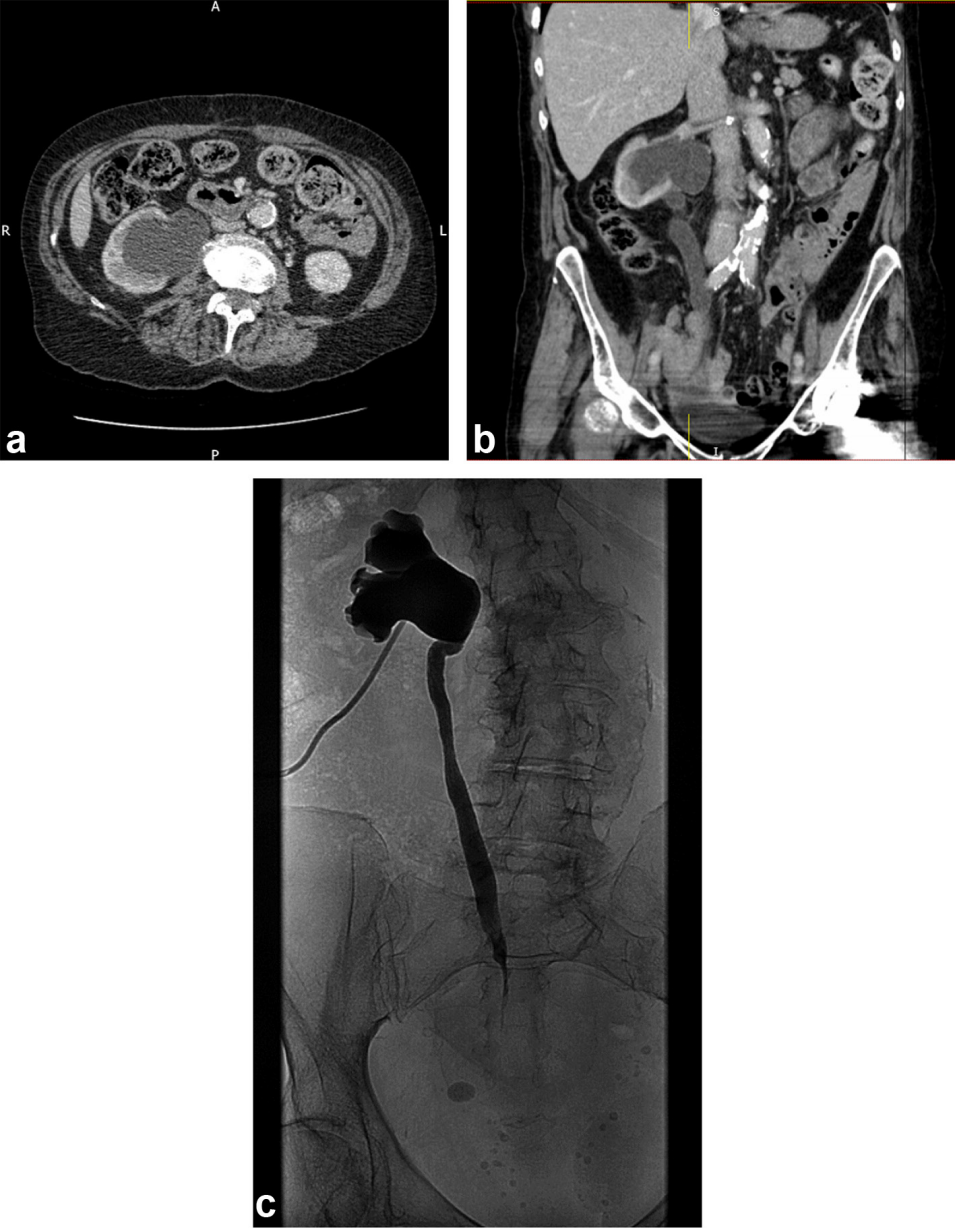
(a) Axial CT section at 6 months post operative, demonstrating marked right hydronephrosis and hydroureter (b). CT coronal reconstruction at 6 months post operative, demonstrating marked right hydronephrosis (1a) and hydroureter (1b). (c).Fluoroscopic renogram performed subsequent to CT (Figure 1) demonstrates right ureteric obstruction secondary to stricturing, likely to be at the site of reimplantation.
